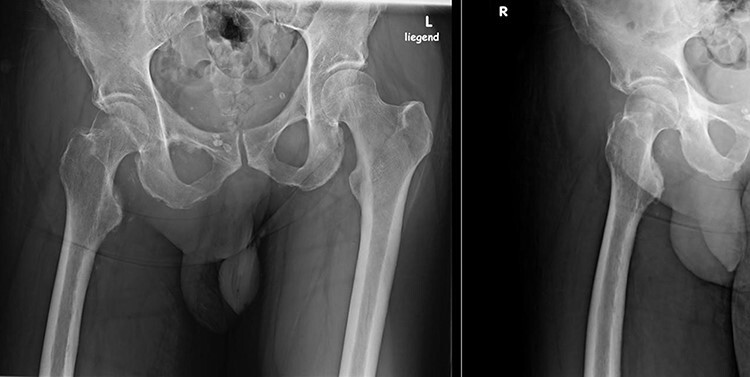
Postoperative pelvic overview radiography and Lauenstein view.
